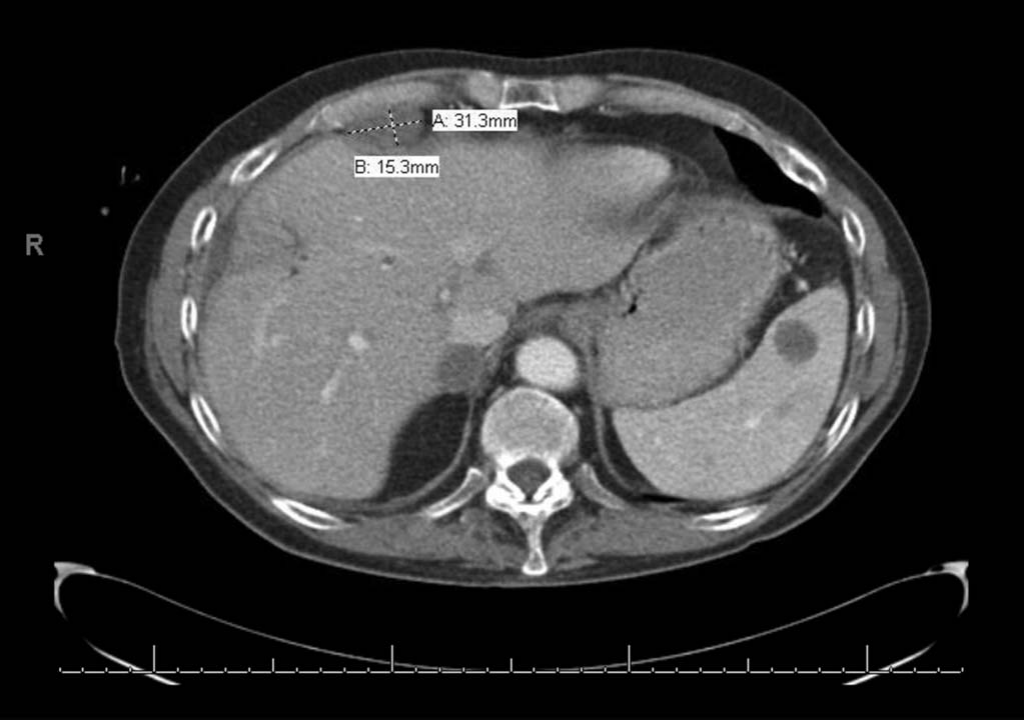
Computed tomography showing retroperitoneal adenopathy.
